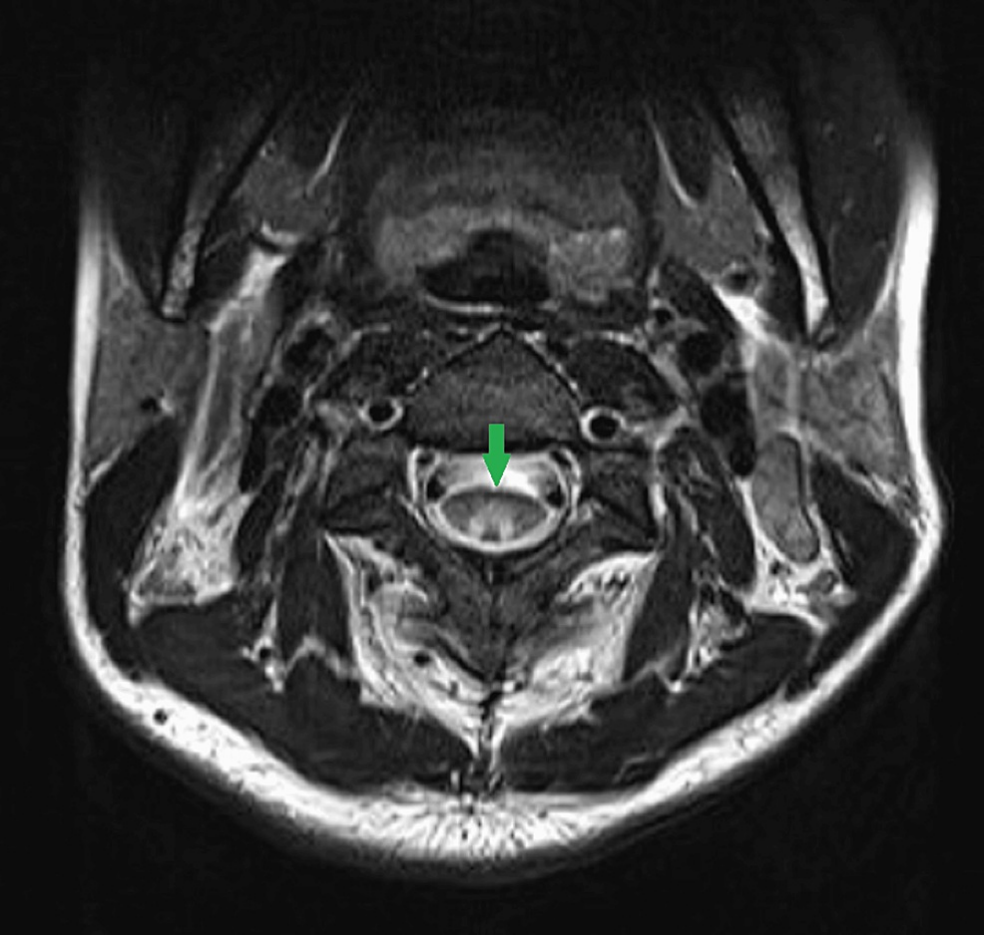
MRI cervical spine T2 weighted image (axial view) Symmetric T2 hyperintense signals within the posterior spinal cord give an inverted V appearance (green arrow).
